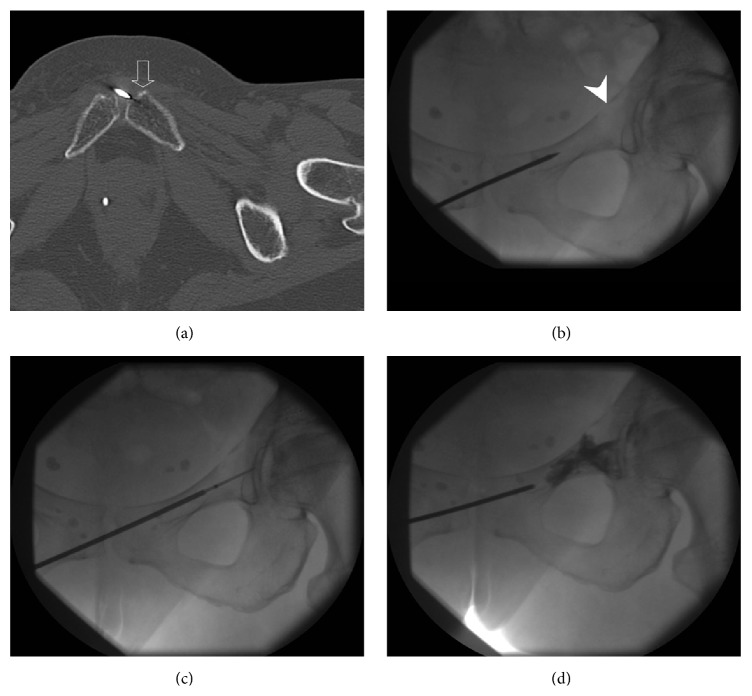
(a) Nonenhanced axial CT image demonstrates the entry point of the retrograde transpubic approach via the pubic tubercle (open arrow). (b)–(d) Anteroposterior fluoroscopic images show the needle guided through the superior pubic ramus toward the lesion (arrowhead) (b), the radiofrequency probe inserted coaxially (c), and the distribution of cement (d) at the end of the procedure.
